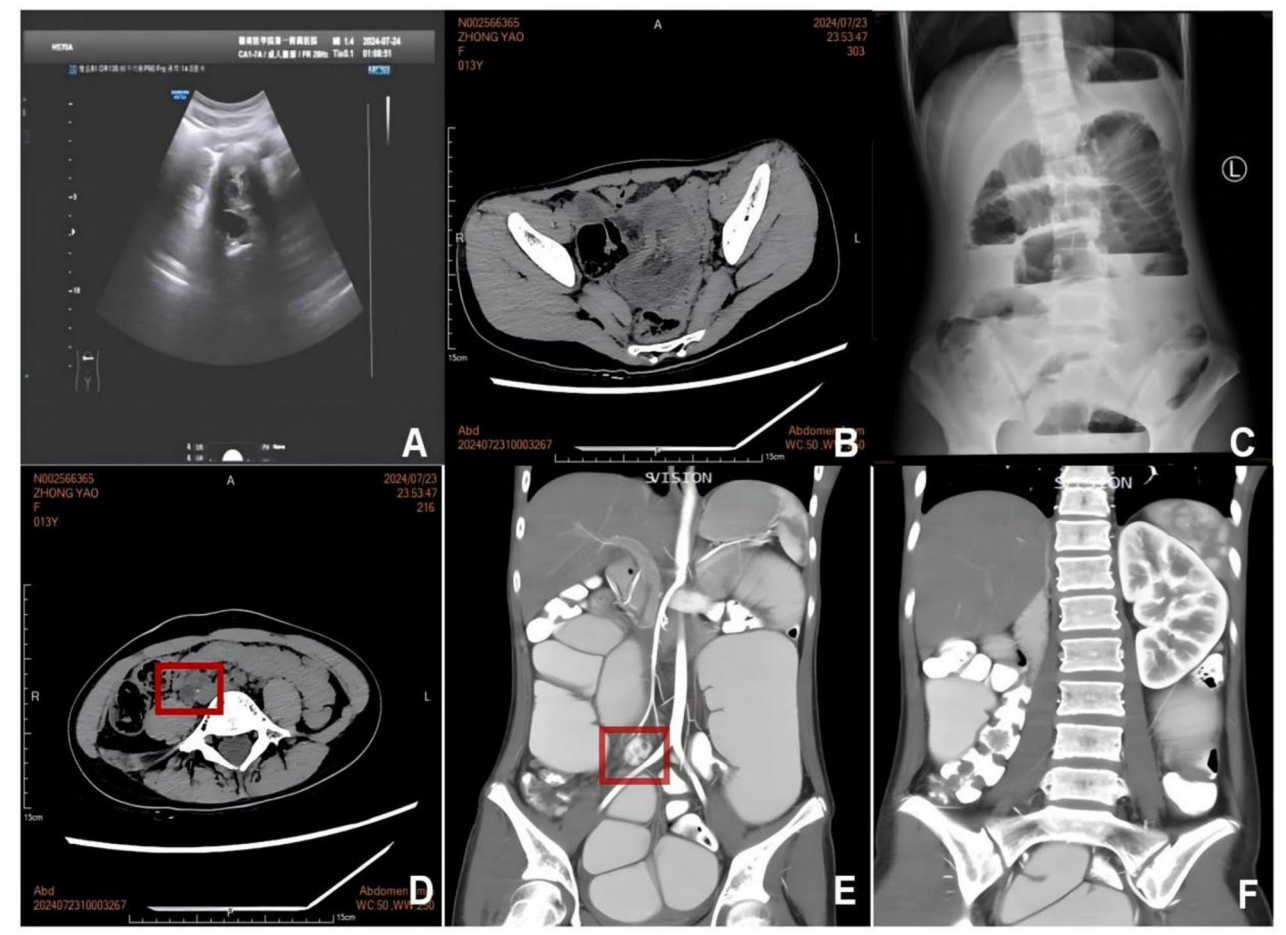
Imaging findings. Ultrasound (A) and Abdominal computed tomography (B) showed intussusception; Plain abdominal X-ray showed intestinal obstruction (C); Abdominal CT showed a complex cystic-solid mass adjacent to the right psoas muscle (marked by red box) (D); Contrast-enhanced abdominal computed tomography angiography demonstrated an atrophic right kidney with multiple cysts (marked by red box) (E) and compensatory enlargement of the left kidney (F)
